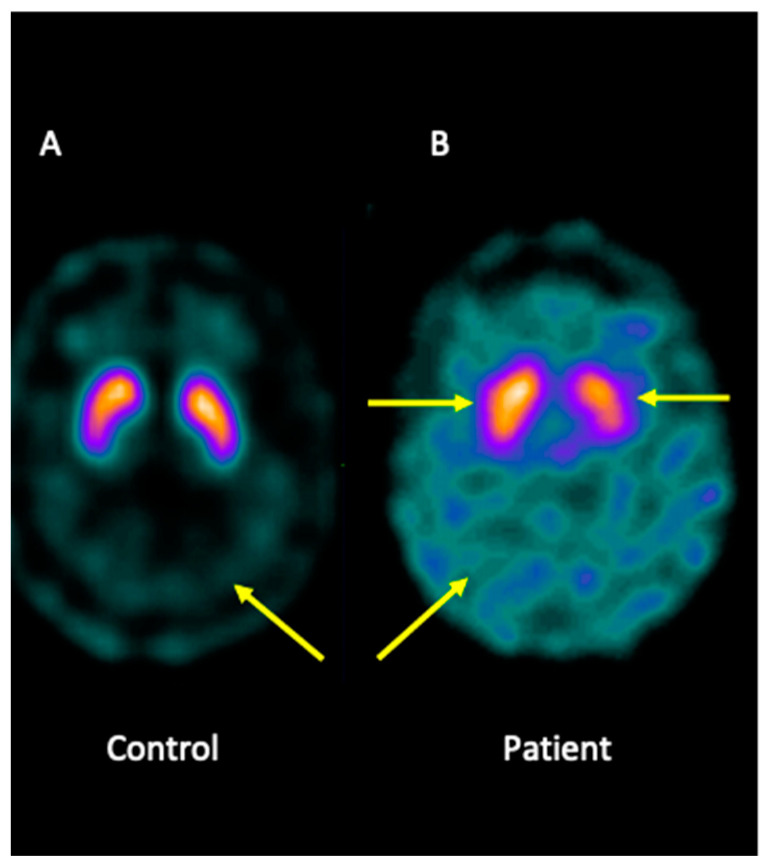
I-123-Ioflupane SPECT image of the brain patient. (A) Healthy control showing both striata with a normal comma shape and sharp borders, presenting low background activity. (B) The patient presented asymmetric striata, with a lower size in the left striata, an oval appearance, and increased background activity.
